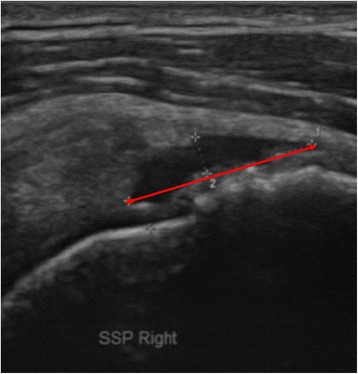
The sonographic image showed the greatest longitudinal tear along the long axis of the rotator cuff tendon and muscle in the modified Crass position. The tear size was measured by the greatest longitudinal tear length (red line) in several longitudinal sections of rotator cuff
